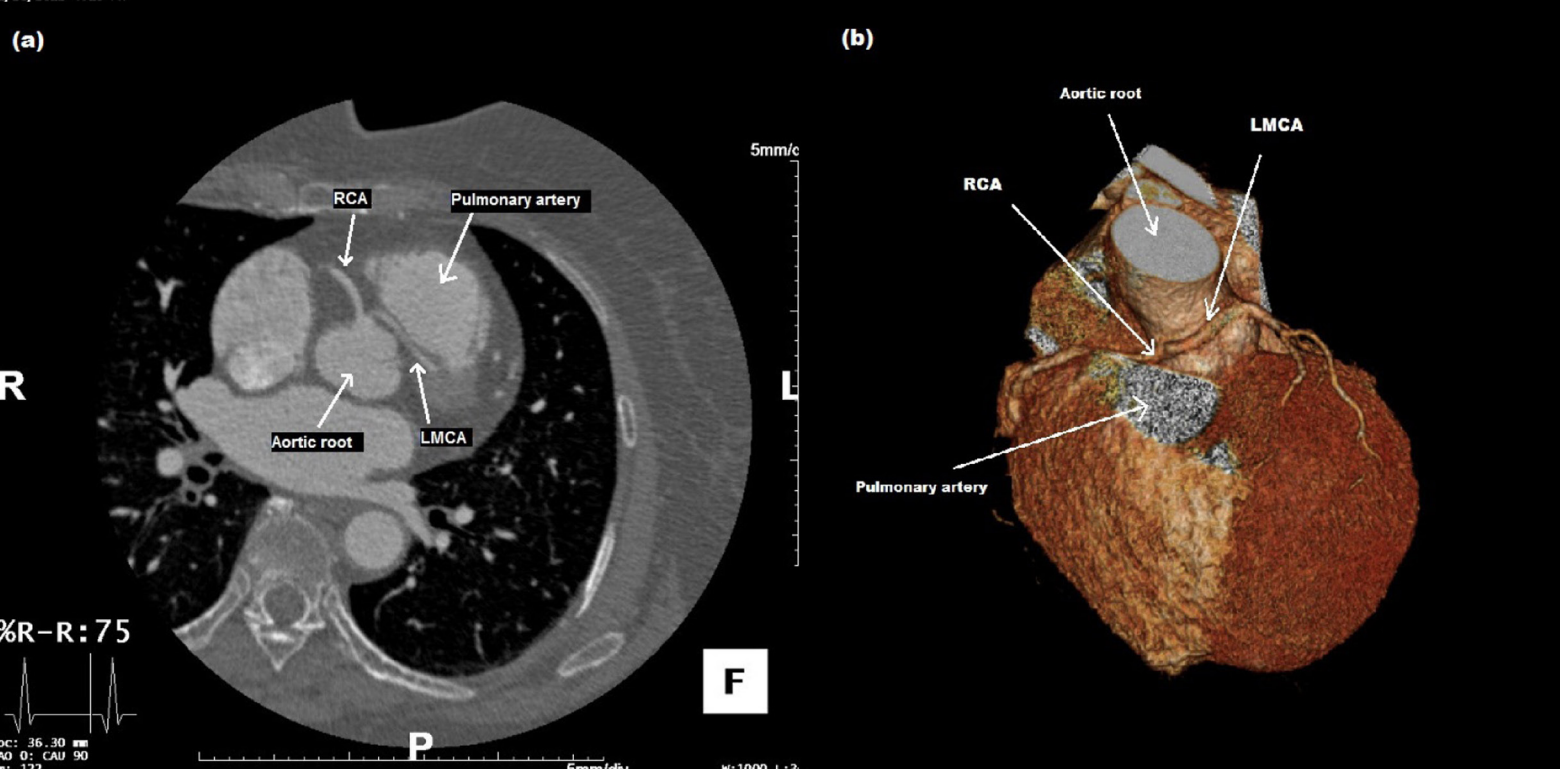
Multidetector Computed Tomography (MDCT); LMCA Coursed between the Aortic Root and Main Pulmonary Artery and Gave off the Left Anterior Descending and Left Circumflex Arteries in Their Normal Position. (a) Female Patient (Case 1), (b) Male Patient (Case 2)
